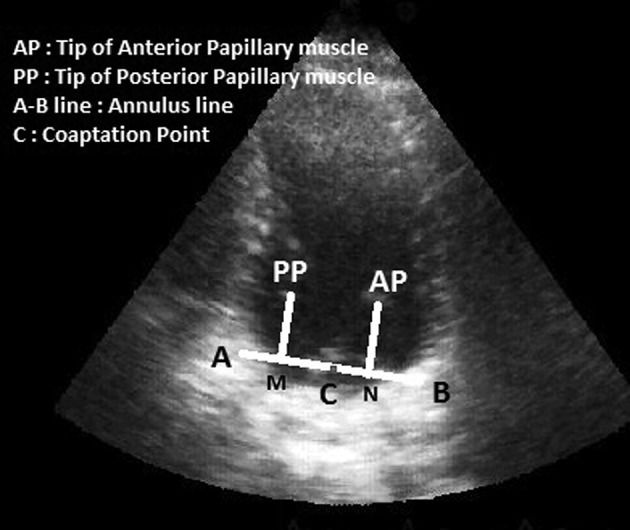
Two-Chamber View in Transthoracic Echocardiography
